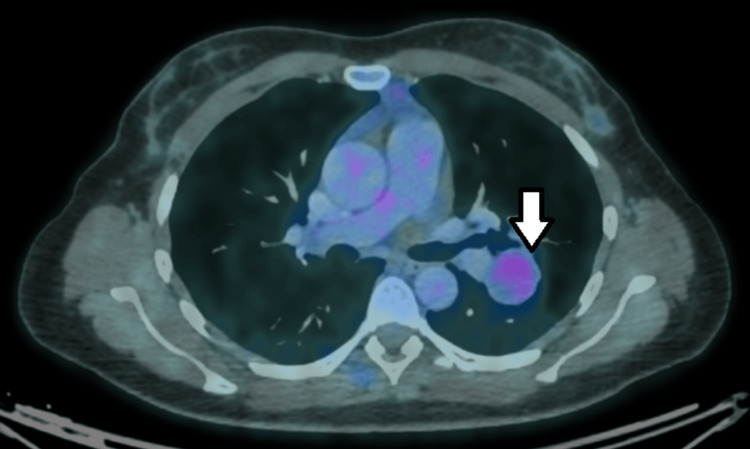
Mild uptake in PET/FDG (SUV = 2.4) of the pulmonary nodule. PET: positron emission tomography; FDG: fluorodeoxyglucose; SUV: standardized uptake value.
